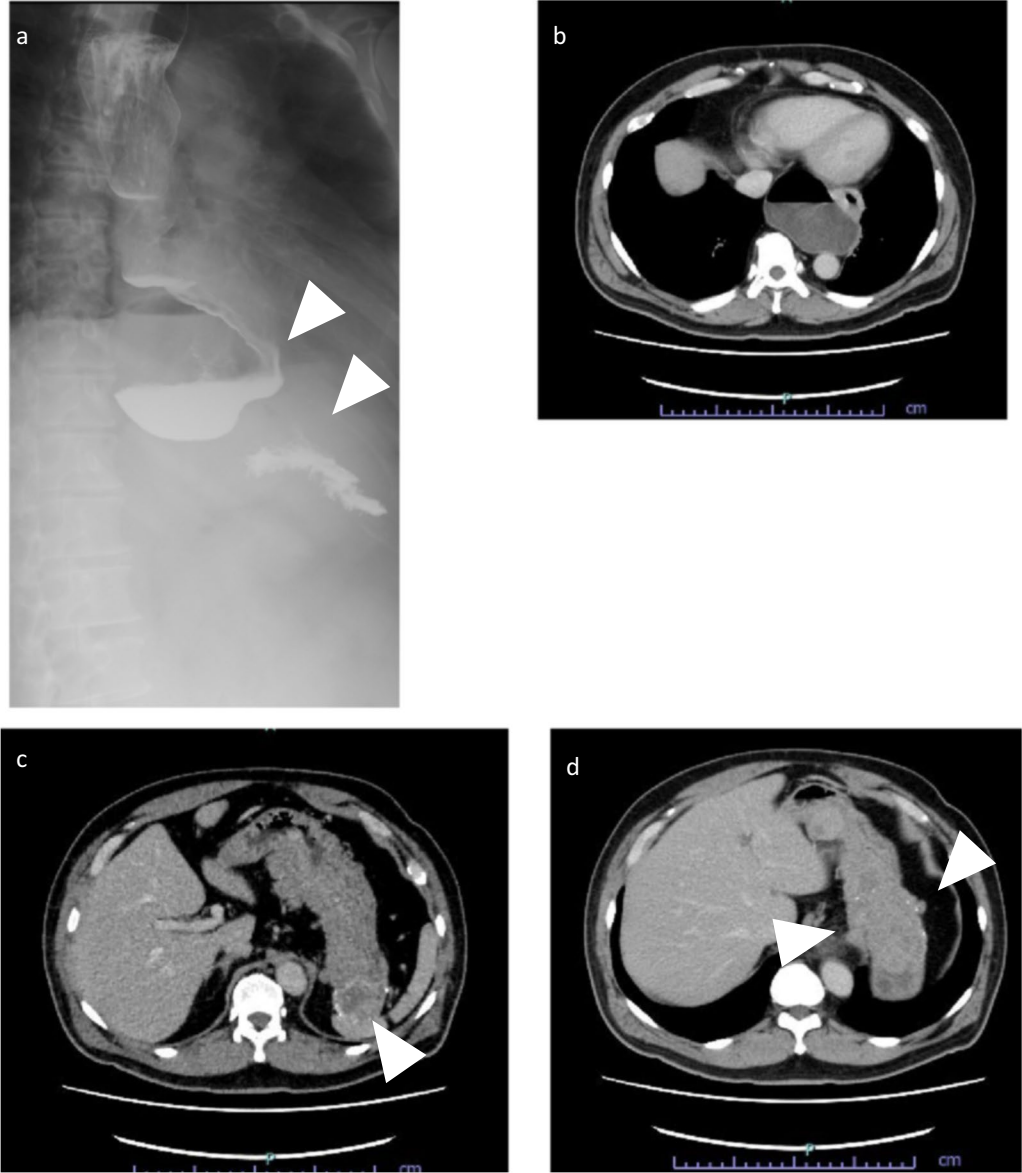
Gastrointestinal series contrast medium did not flow into the stomach smoothly (white arrow heads) because of the large esophageal diverticulum (a). CT images of the esophageal diverticulum (b), gastric large GIST (white arrow head) (c) and multiple GISTs (white arrow heads) (d)
